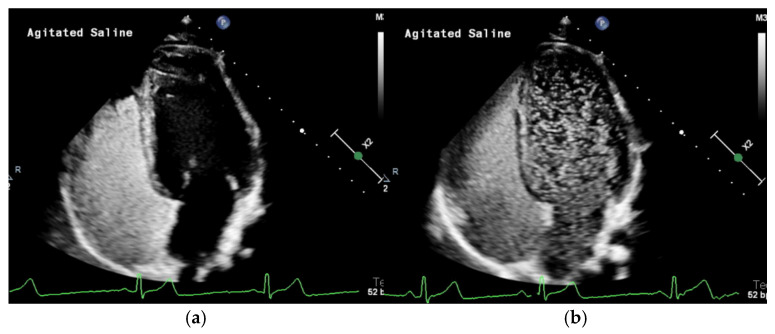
(a) Transthoracic contrast echocardiography with agitated saline (TTCE) demonstrates the opacification of the right heart with microbubbles. (b) TTCE in the same patient eight cardiac cycles later showing a grade 3 shunt from multiple large PAVMs.
